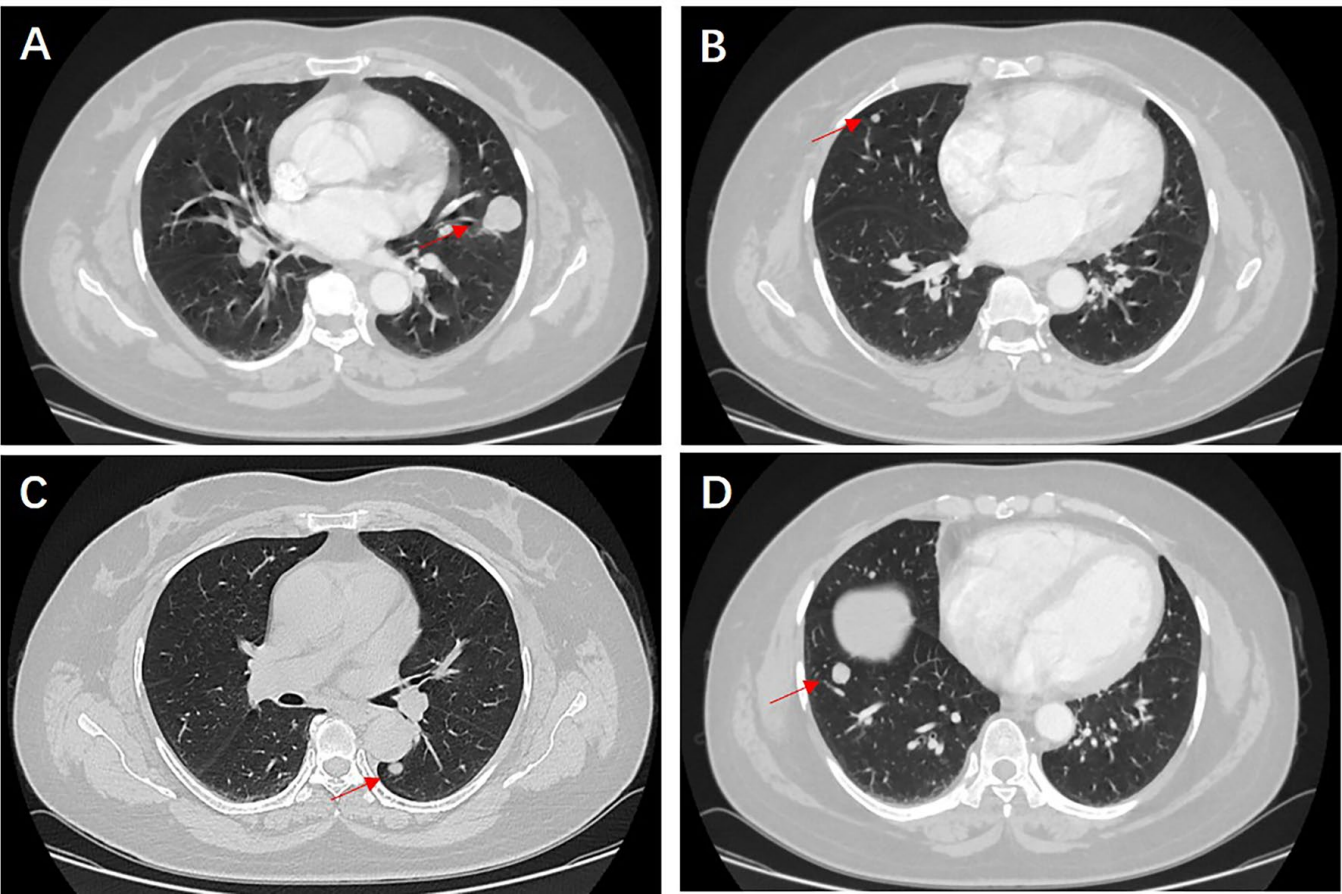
Chest CT of multiple PSP. This is a rare case of multiple PSP. The patient’s physical examination revealed the presence of multiple nodules in both lungs. A chest CT scan confirmed the existence of a total of 4 circular pulmonary nodules, distributed as follows: 1 in the upper lobe of the right lung, 1 in the lower lobe of the right lung, 1 in the upper lobe of the left lung, and 1 in the lower lobe of the left lung. The largest nodule, measuring 26 mm in diameter, was surgically excised from the left upper lobe and subjected to pathological analysis, which confirmed the diagnosis of PSP. Consequently, the final diagnosis was determined to be multiple PSP, and the patient is currently undergoing follow-up care.
